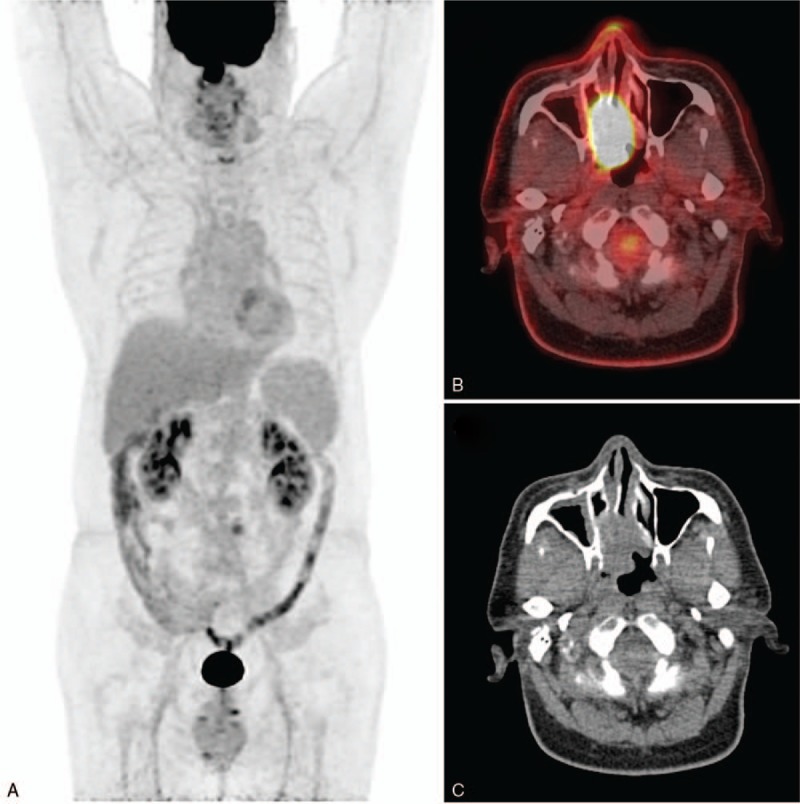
FDG PET/CT at baseline assessment. PET MIP (A), axial fusion image (B) and axial CT (C) showed a mass with intense FDG uptake in the right nasal cavity with size of 4.1×2.3 and SUVmax of 26.0.
